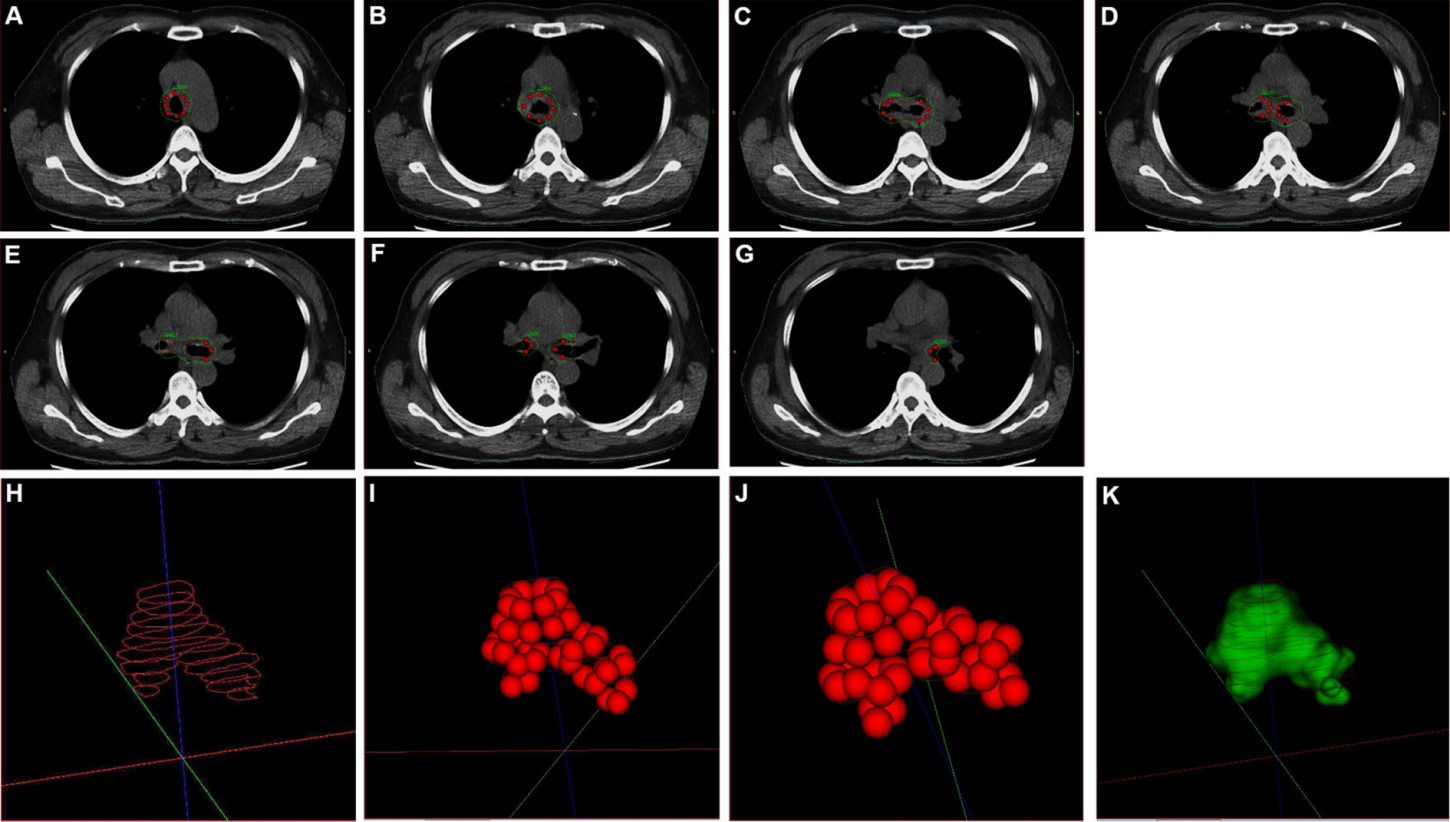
The number and distribution of seeds in plan before placement. (A–G) The seeds distribution and isodose distribution in thoracic computerized tomography (CT) scans. (H) Planning target volume (PTV) in three-dimension (3D). (I, J)
